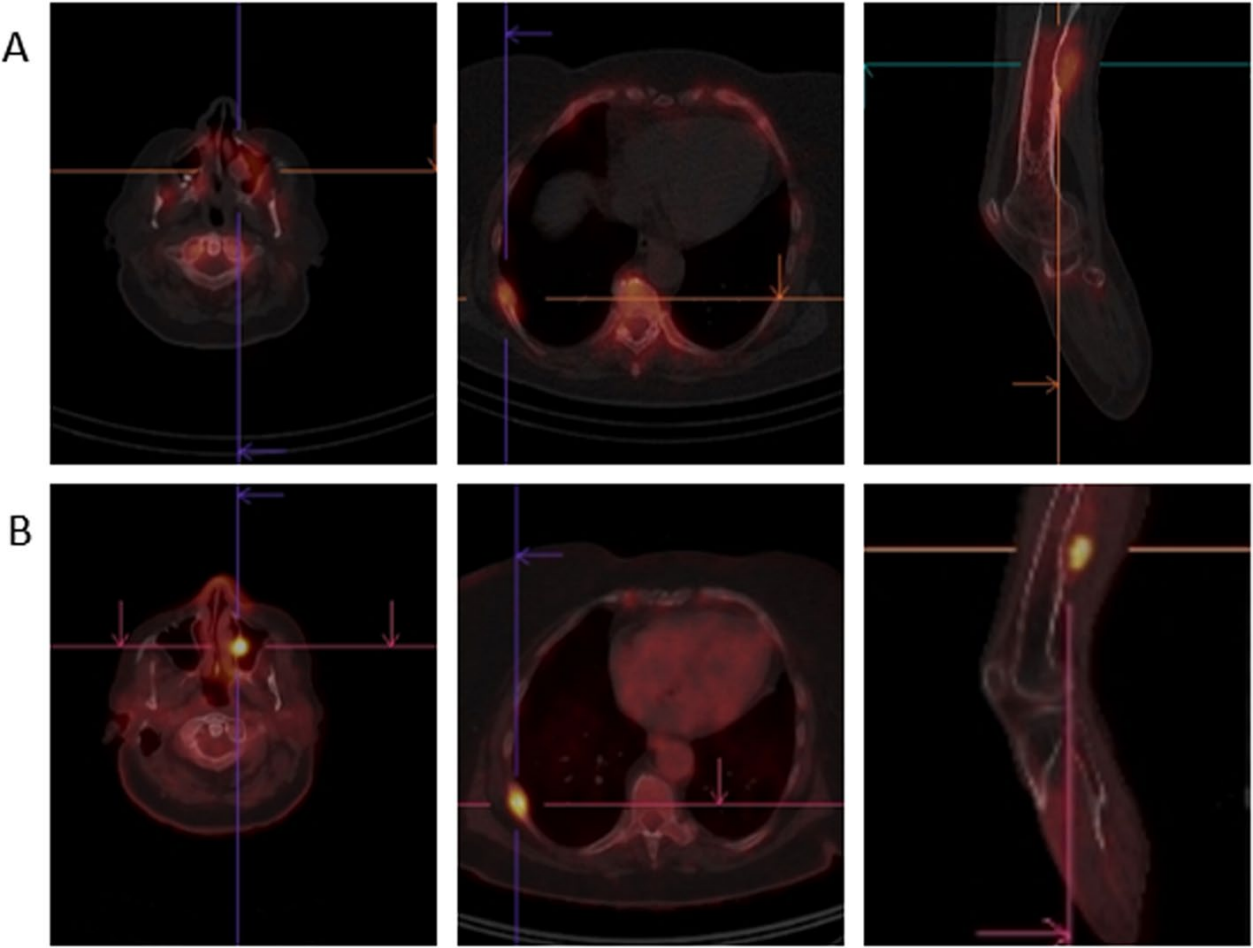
Comparison of the presentation of 3 bone lesions on bone SPECT/CT (upper row; A) and ^18^F-FDG-PET/CT (lower row; B), respectively, in the skull (left maxillary sinus), a rib and the right femur
